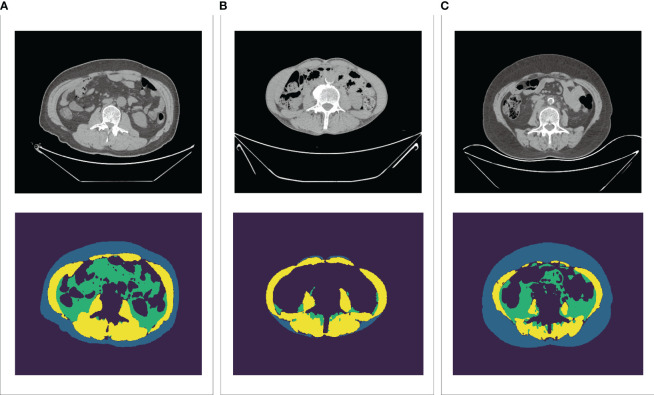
U-Net-based segmentation of body composition using CT images. Yellow=SMA, Blue=SATA, Green=VATA. (A) Representative of patients with high SMA and TATA. (B) Representative of patients with high SMA and low TATA. (C) Representative of patients with low SMA and high TATA. SMA, skeletal muscle area; SATA, subcutaneous adipose tissue area; VATA, visceral adipose tissue area, TATA, total adipose tissue area.
